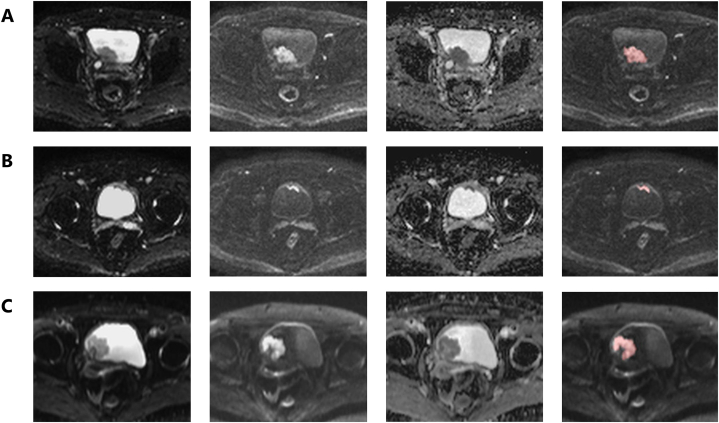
Three representative cases of bladder cancer (BC) in the external test dataset. Left to right: Diffusion-weighted images (b = 0 s/mm^2^), diffusion-weighted images (b = 1000 s/mm^2^), apparent diffusion coefficient map, and manually segmented region of interest in the BC. (A) Male patient in his 60s with MIBC. Pelvic MRI revealed an exophytic tumor without a stalk on the posterior bladder wall. All six radiologists assigned a VI-RADS score of 4. The output of the ViT-based diagnostic model was 0.976, which is a strong indicator of MIBC. (B) Male patient in his 60s with NMIBC. Pelvic MRI revealed a slightly exophytic tumor without a stalk on the anterior wall of the bladder. Four radiologists assigned a VI-RADS score of 4, and the other two radiologists assigned a VI-RADS score of 3. The output of the ViT-based diagnostic model was 0.301, which is indicative of NMIBC. (C) Female patient in her 70s with NMIBC. Pelvic MRI revealed an exophytic tumor with a stalk on the right lateral wall of the bladder. All six radiologists assigned a VI-RADS score of 2. The output of the ViT-based diagnostic model was 0.515, which was more suggestive of MIBC. BC: Bladder cancer; MIBC: Muscle-invasive bladder cancer; NMIBC: Non-muscle invasive bladder cancer; VI-RADS: Vesical Imaging Reporting and Data System; and ViT: Vision Transformer.
